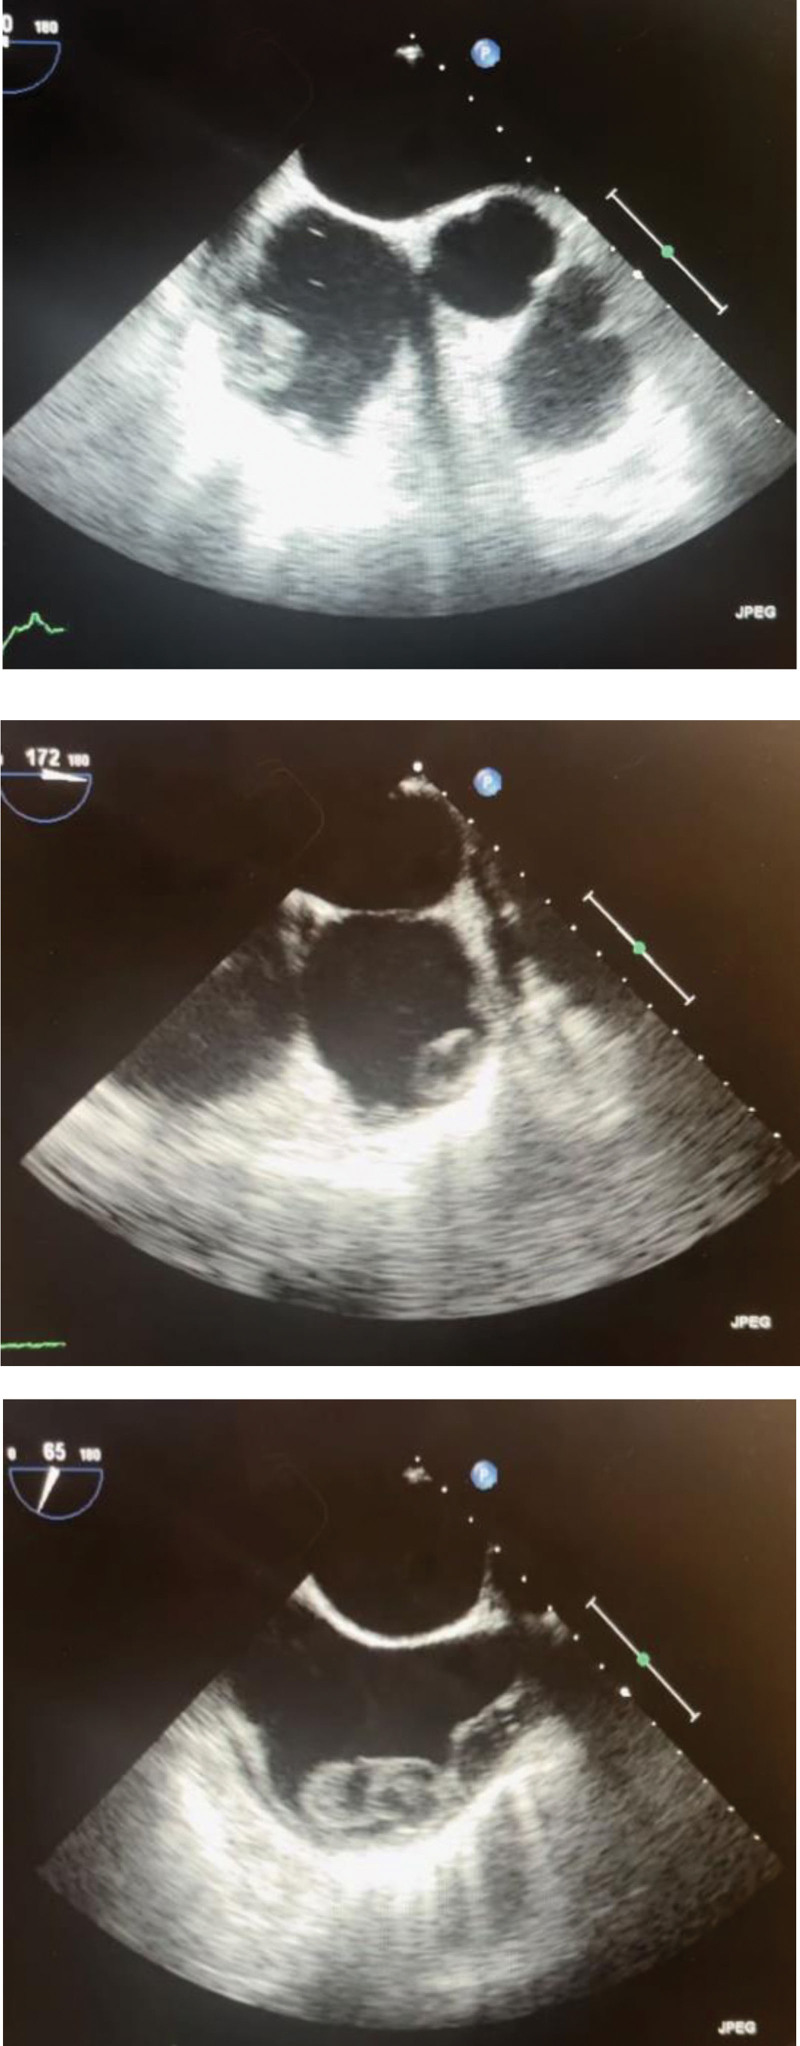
Images showing the mass in the RA from multiple views using TEE. RA = right atrium, TEE = transesophageal echocardiography.
